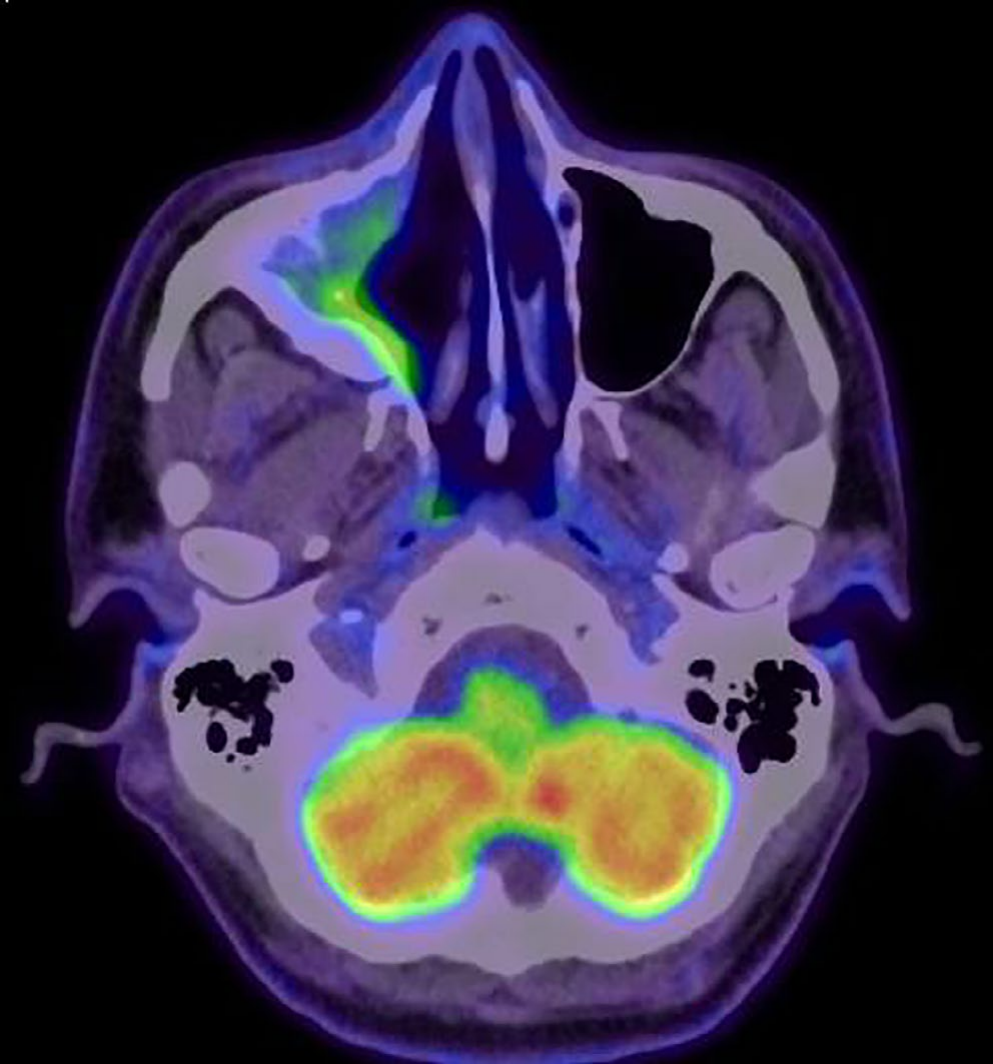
FDG PET CT scan showing axial cut of the sinus with metabolic activity limited to the right maxillary sinus walls
